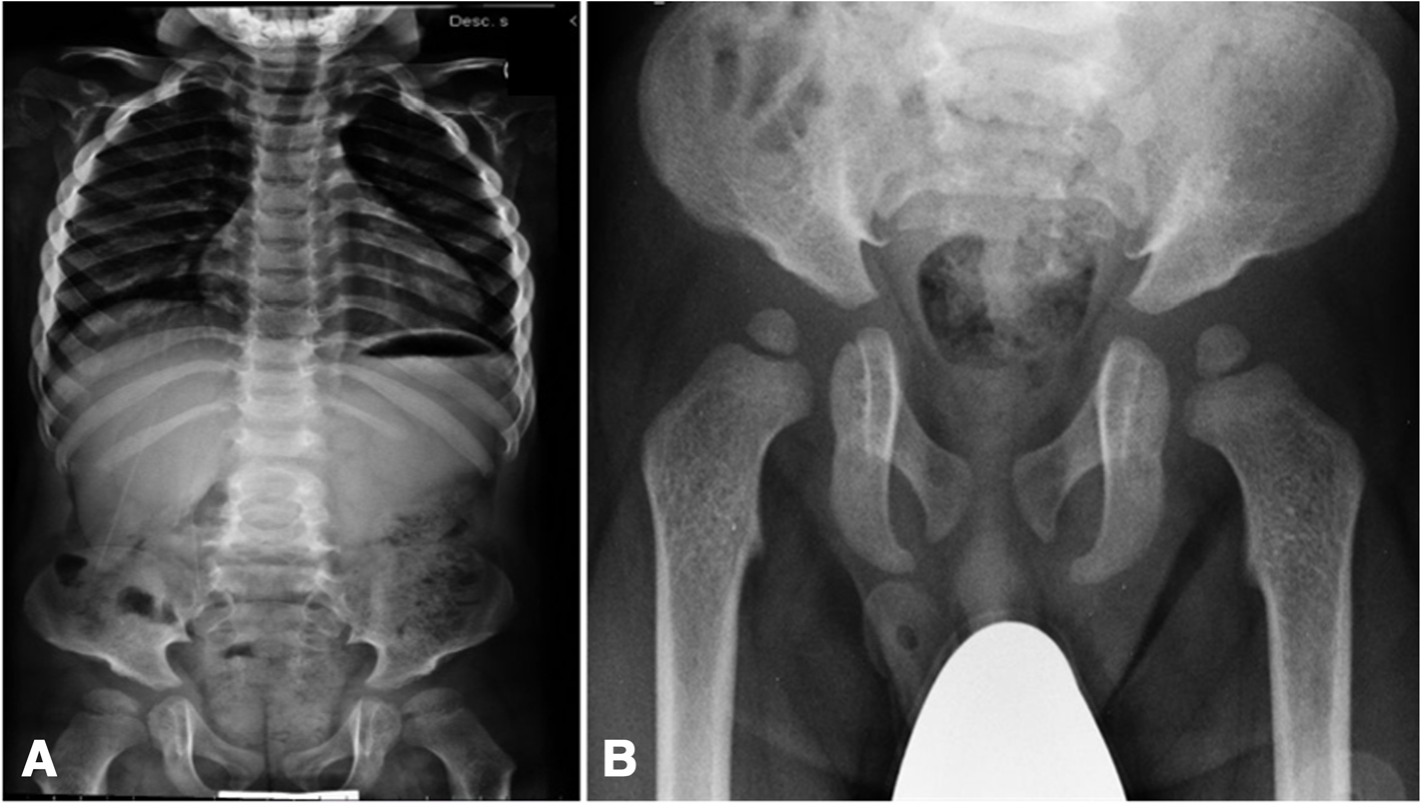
MPS IH. X-ray evidence of dysostosis multiplex. a Thickening of the ribs in a 3-year-old patient and b hip dysplasia in another patient aged 18 months
